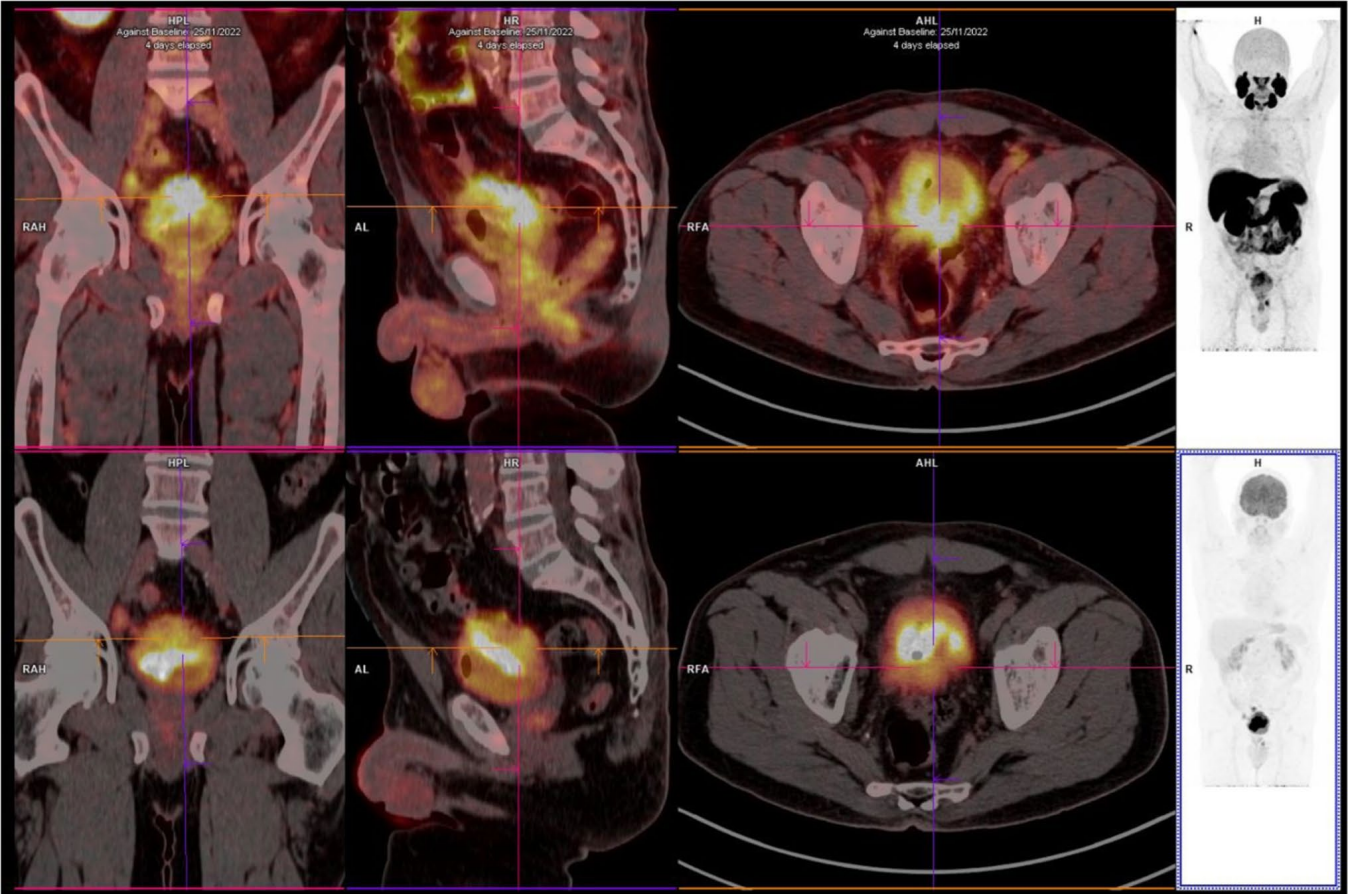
58-year-old, male, T4N1M1, poorly differentiated carcinoma with extensive areas of squamous differentiation. From left to right: coronal, sagittal, axial, and maximum intensity projection [^18^F]PSMA-1007 (top row) and post-diuretic [^18^F]FDG (bottom row) PET/CT images of a patient with muscle-invasive bladder cancer (patient 1, Table 1). Note that an extensive bladder lesion is more clearly identified on [^18^F]PSMA-1007 than on [^18^F]FDG images, in which the lesion cannot be distinguished from urinary excretion of the radiotracer, even after diuretic administration.
